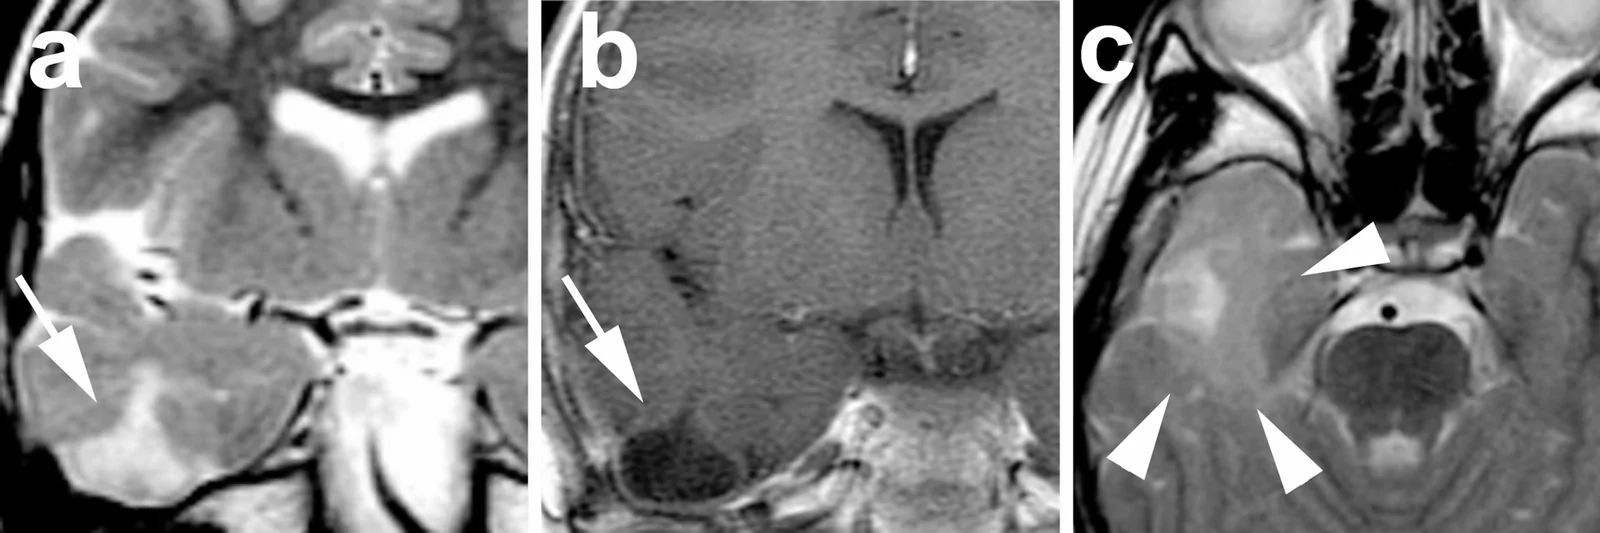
A 7-year-old girl with a ganglioglioma presenting with facial spasms and left arm automatisms. Coronal T2-weighted image (a) and contrast-enhanced coronal T1-weighted image (b) show a cystic mass in the right temporal cortex without appreciable contrast enhancement (arrows). On the axial T2-weighted image (c), ill-defined hyperintensity is observed in the adjacent cortex and white matter (arrowheads), corresponding to focal cortical dysplasia associated with the tumor
